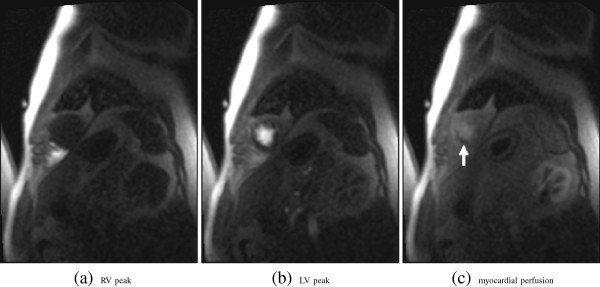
Example images from a first-pass gadolinium-enhanced myocardial perfusion MRI study (patient 5, stress, apical slice): RV enhacement peak (a), LV enhancement peak (b) and myocardial perfusion (c). Note, the hypointense region in the perfused myocardium (c) indicates a reduction in blood flow, i.e. the medical condition that needs to be quantified for the assessment.
